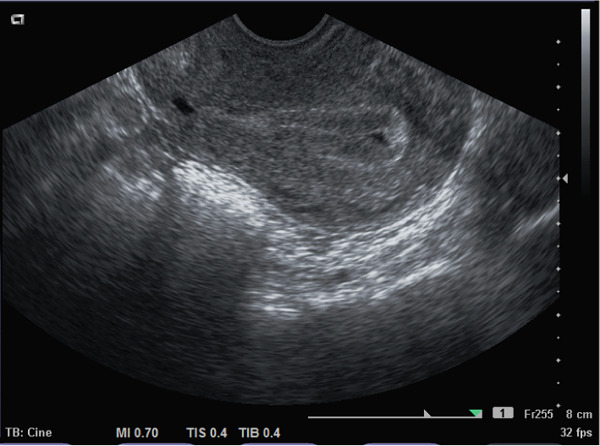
Transvaginal sonogram image at midcycle showing cesarean section isthmocele (CSI) and endometrial cavity fluid.
